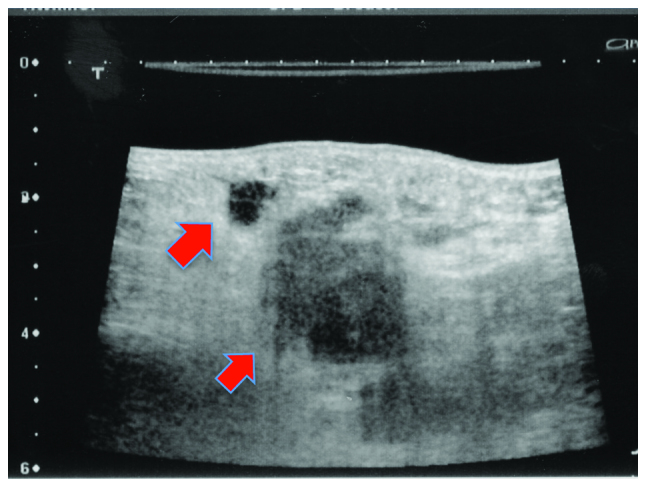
Breast ultrasonography of the axillary lesion. A minimum of 20 irregular masses of dispersed sizes were observed surrounding the suspected metastatic left axillary lymph node. The smallest and greatest diameters observed were 5 and 35 mm, respectively.
